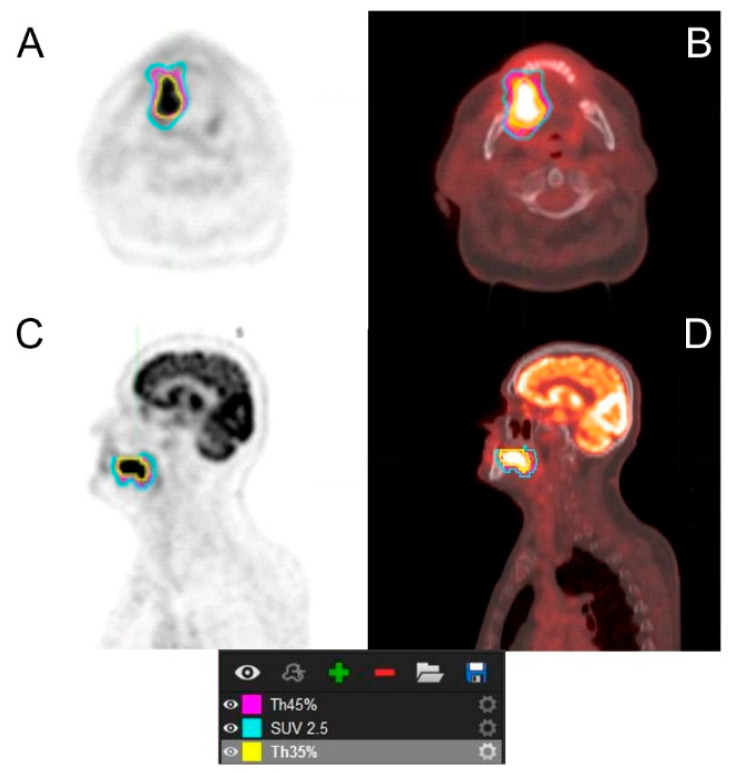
An example of segmented image according to the chosen segmentation method. (A) PET images in transverse plane, (B) fused PET/CT images in transverse plane, (C) PET image in sagittal plane, (D) fused PET/CT images in sagittal plane.
